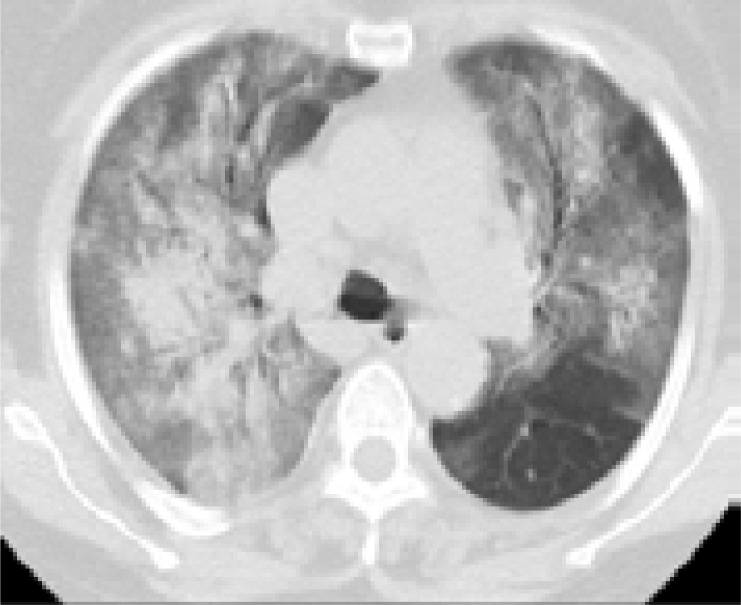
I) A 76-year old female patient with COPD history had the complaints of cough and joint pain for 10 days. No pathological finding was found in chest CT image of the patient which was scanned 7 days before application to the hospital. She applied due to excessive increase of dyspnea complaint. Opacities of peripheral subpleural parenchymal ground glass densities and consolidations were found in all lobes and segments of both lungs suggesting severe COVID-19 in chest CT image of the patient. (They are indicated in black oval shapes)
